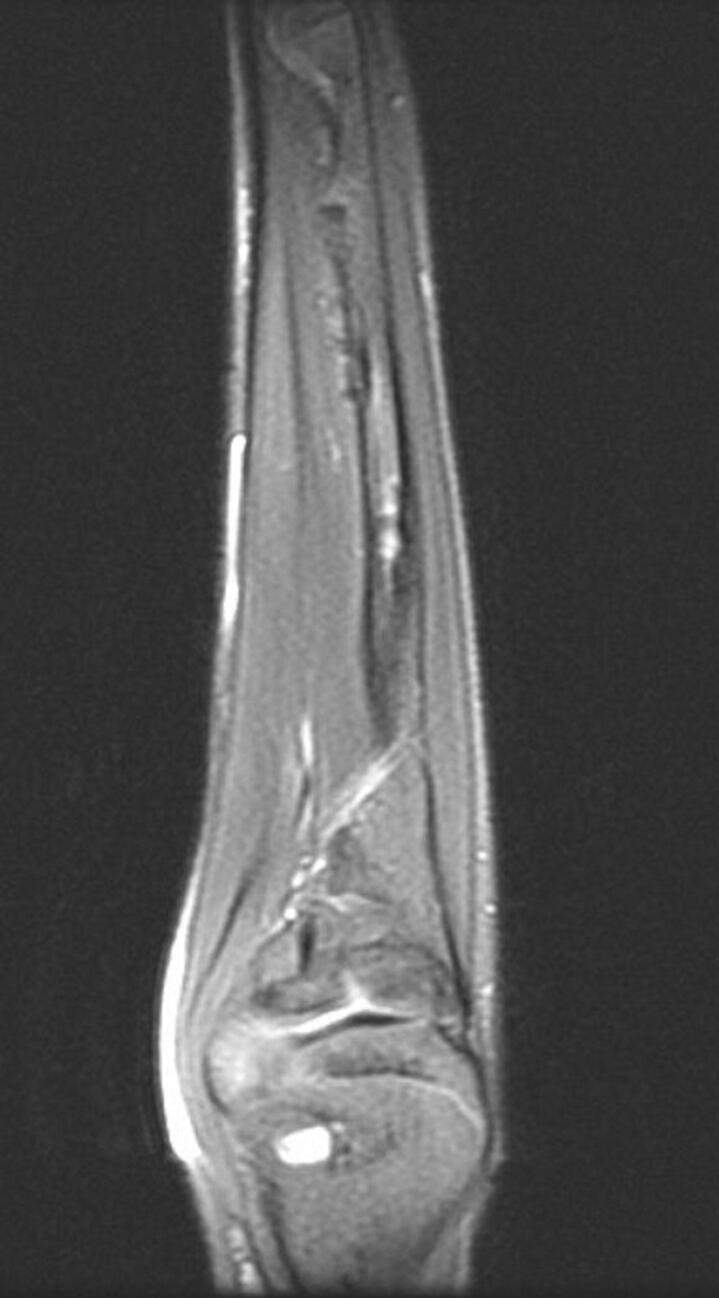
Postoperative MRI indicates the total removal of the FCD (sagittal view).
